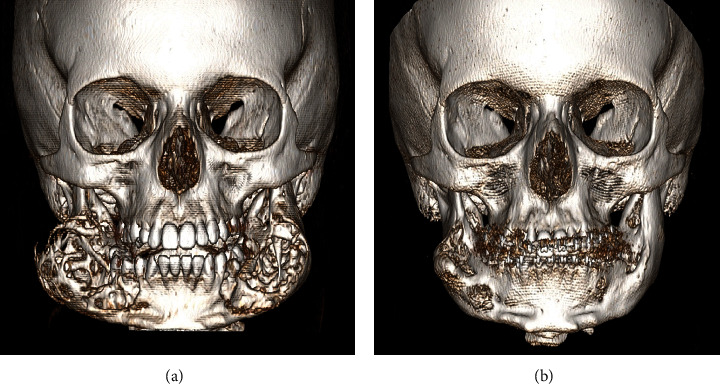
3D reconstruction of computed tomography at start of treatment (a) and after 35 months of treatment (b). Notice the massive lateral expansion of the lesions as well as severe cortical thinning at start of treatment. After 35 months, lesions were reduced in size and largely ossified facilitating future corrective surgery.
